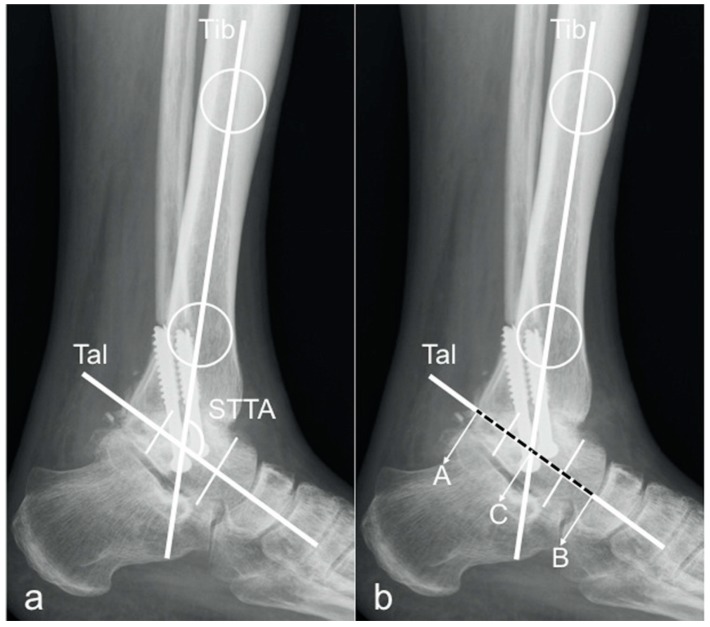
Method 2 for STTA and mTT-ratio measurement. Lateral ankle radiograph demonstrating Method 2: Tib = longitudinal axis of the tibia, created by connecting two points in the middle of the proximal and distal tibial shaft. Tal = or a line drawn connecting the midpoints of a line bisecting the talar neck and another line bisecting the talar body. Figure (a) depicts measurement of the sagittal tibio-talar angle (STTA). Figure (b) shows measurement of the mT-T ratio.
